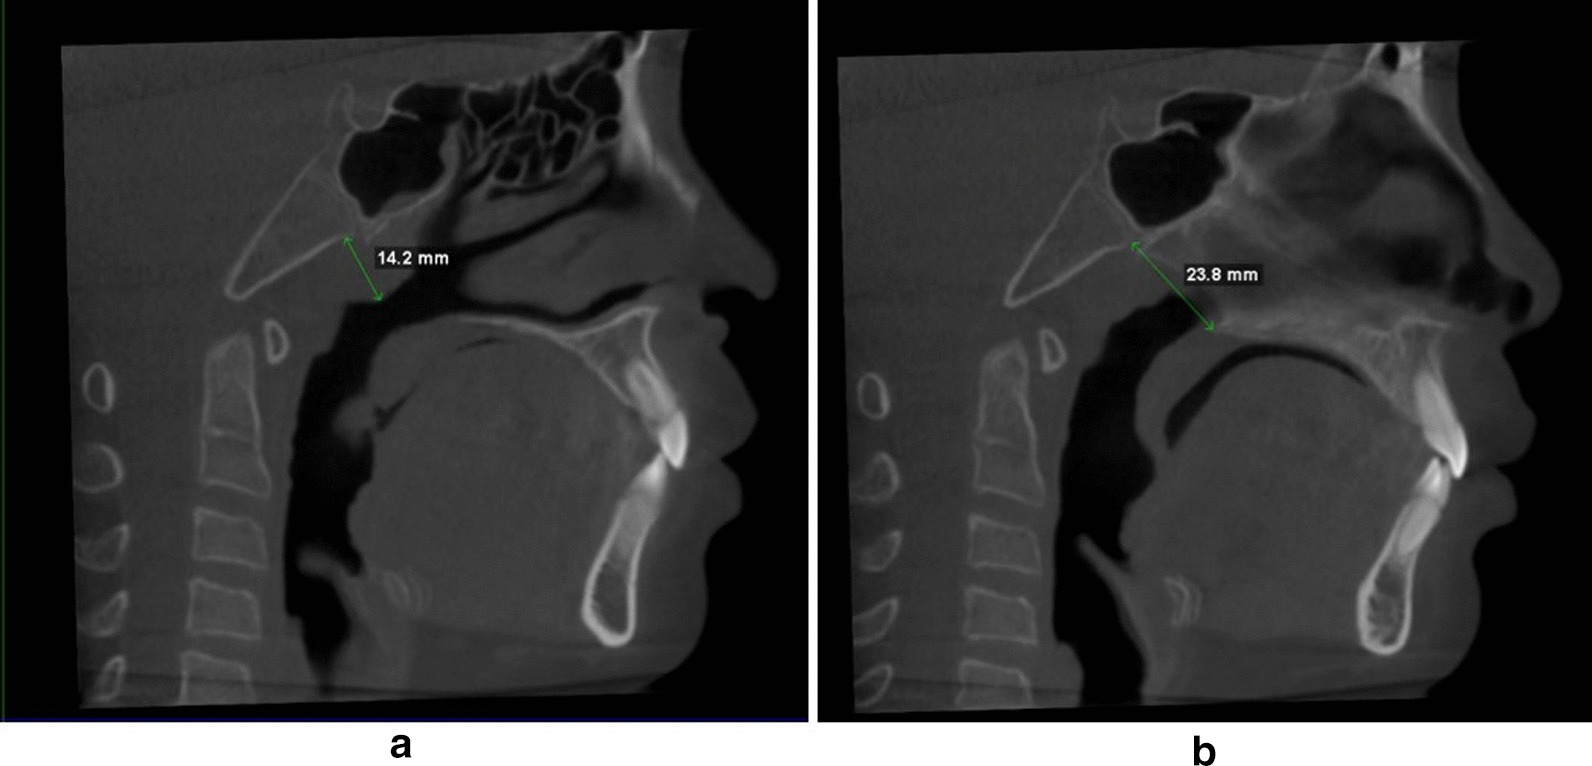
The measurement of AN ratio on CBCT images. a A, perpendicular distance from the maximal convexity of the adenoid identifying by scrolling through the sagittal slice that showed maximal convexity of the adenoid (where the intersecting axial view also showed maximal convexity) to the anterior margin of the basiocciput. b N, distance between the posterosuperior point of the hard palate and the anteroinferior point of the spheno-occipital synchondrosis on the mid-sagittal plane
